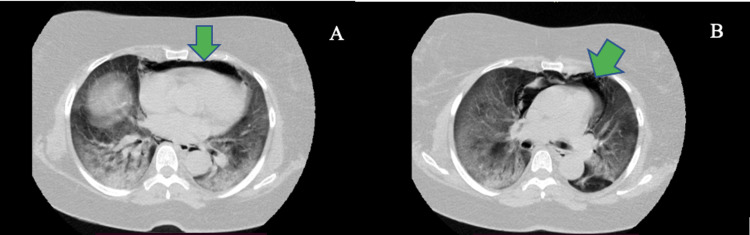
A, B Axial computed tomography image showing pneumomediastinum (green arrows).
